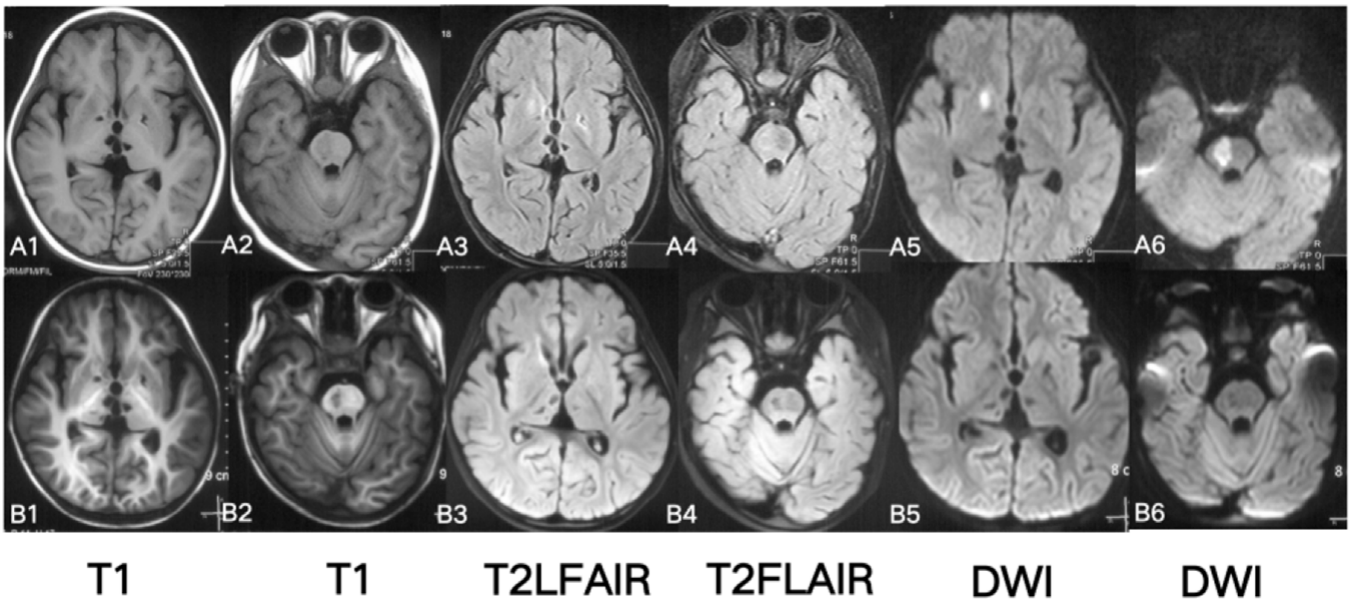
Brain MRI A1-6: at 2 years and 4 months of age (2 weeks of disease duration after disease onset): T2WI, T2-FLAIR, DWI high, and T1WI low signals in the right putamen and brainstem; T2WI, T2-FLAIR, and T1WI low signals in the bilateral thalamus and basal ganglia. B1-6: at 2 years and 5 months old (1.5 months after disease onset): T2, T2-FLAIR high signals in the right putamen and right brainstem disappeared.
